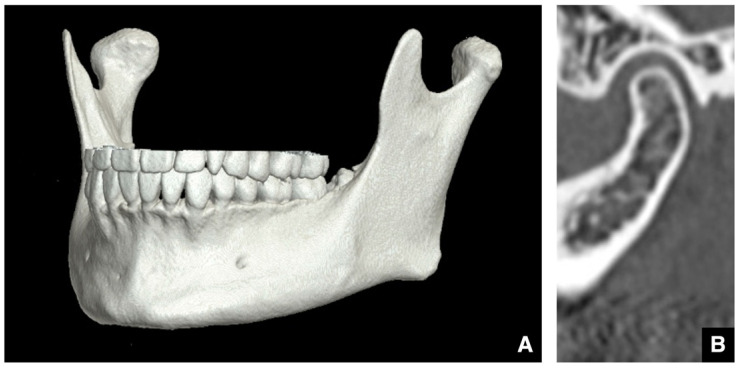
CT images of normal temporomandibular joints. (A) A 3D reconstructed image created from MDCT image data (slice thickness, 0.5 mm) shows normal mandibular condyles. (B) Corrected sagittal MDCT image shows a normal temporomandibular joint. Permission has been obtained to publish all figures. Created from CT data.
